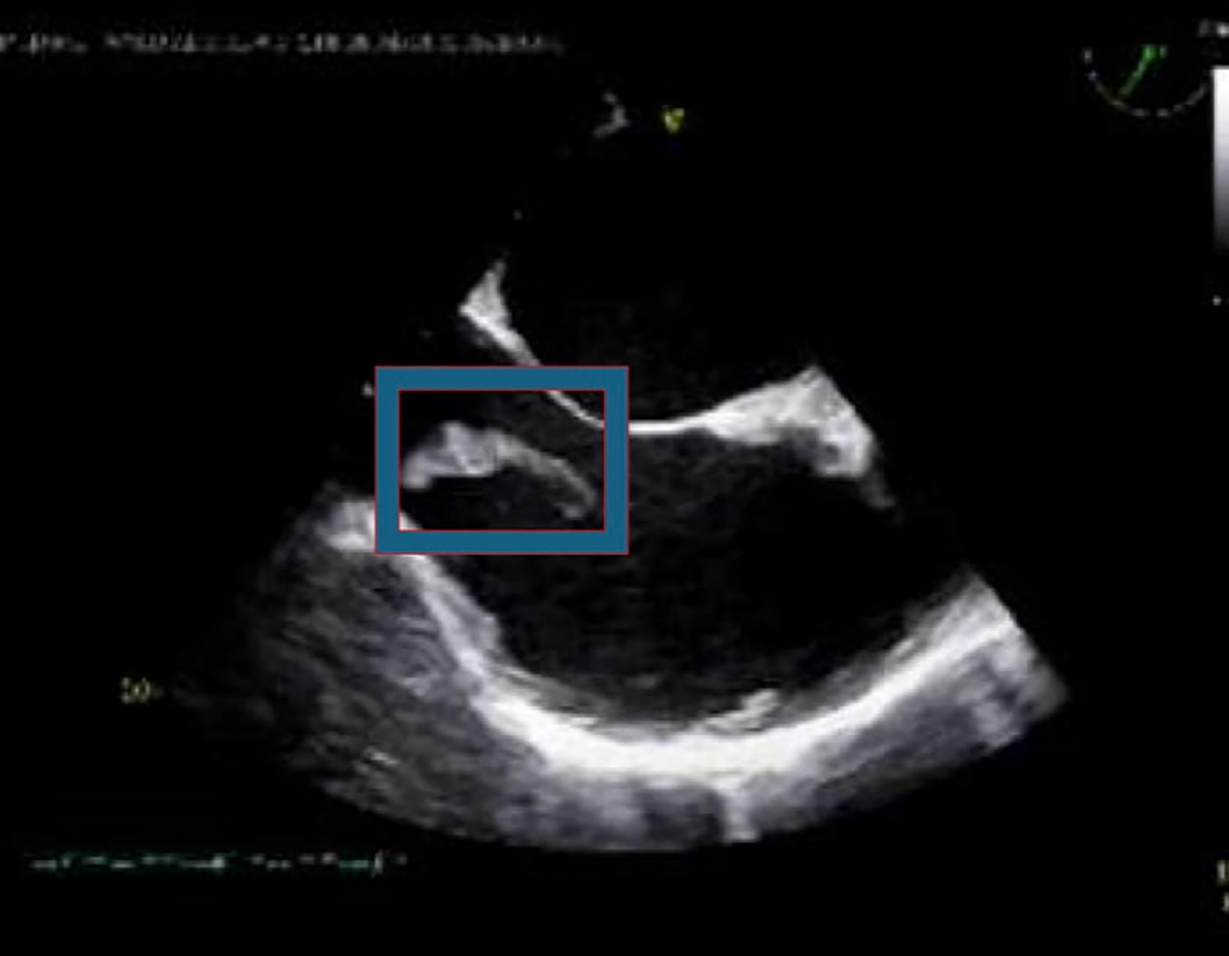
Transesophageal echocardiography showing vegetations.
